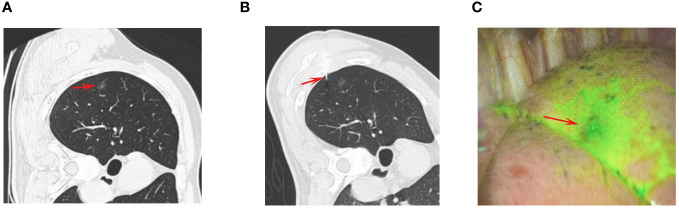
The procedures of CT-guided IG localization. (A) The chest CT showed a GGN (arrow) at the right upper lobe. (B) The puncture needle (arrow) was sent into the lung and near the GGN for IG localization. (C) The IG (arrow) could be visualized during the VATS procedure.
